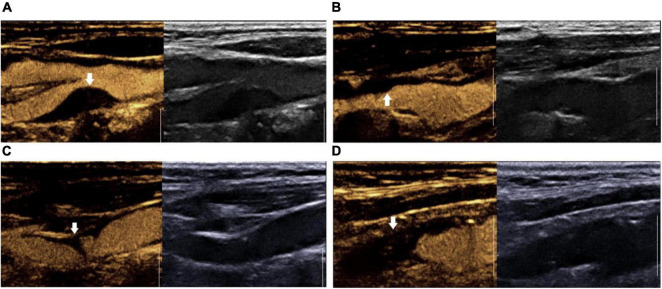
Surface morphology grading of contrast-enhanced ultrasound plaque. (A) Grade I: smooth plaque surface group; (B) Grade II: irregular plaque surface group. (C) Grade III: ulcerated plaque group; (D) Grade IV: carotid artery occlusion group.
